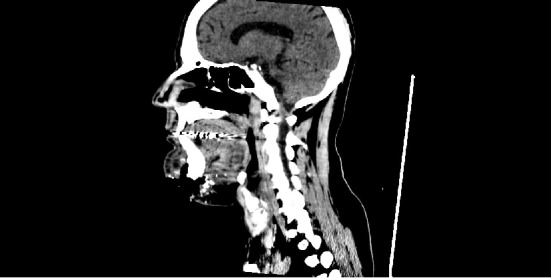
Sagital section of computed tomography contrast enhanced, revealing extensive damage to the suprahyoid soft tissues.
